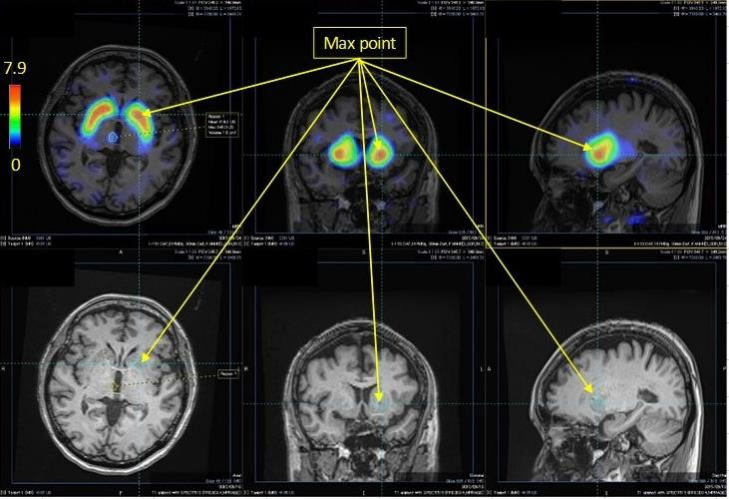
An example of a specific binding ratio (SBR) image of the striatum. DAT Dopamine transporter accumulation is localised in the striatum, as revealed by overlapping MRI the magnetic resonance image, and the maximum SBR value is 7.9
